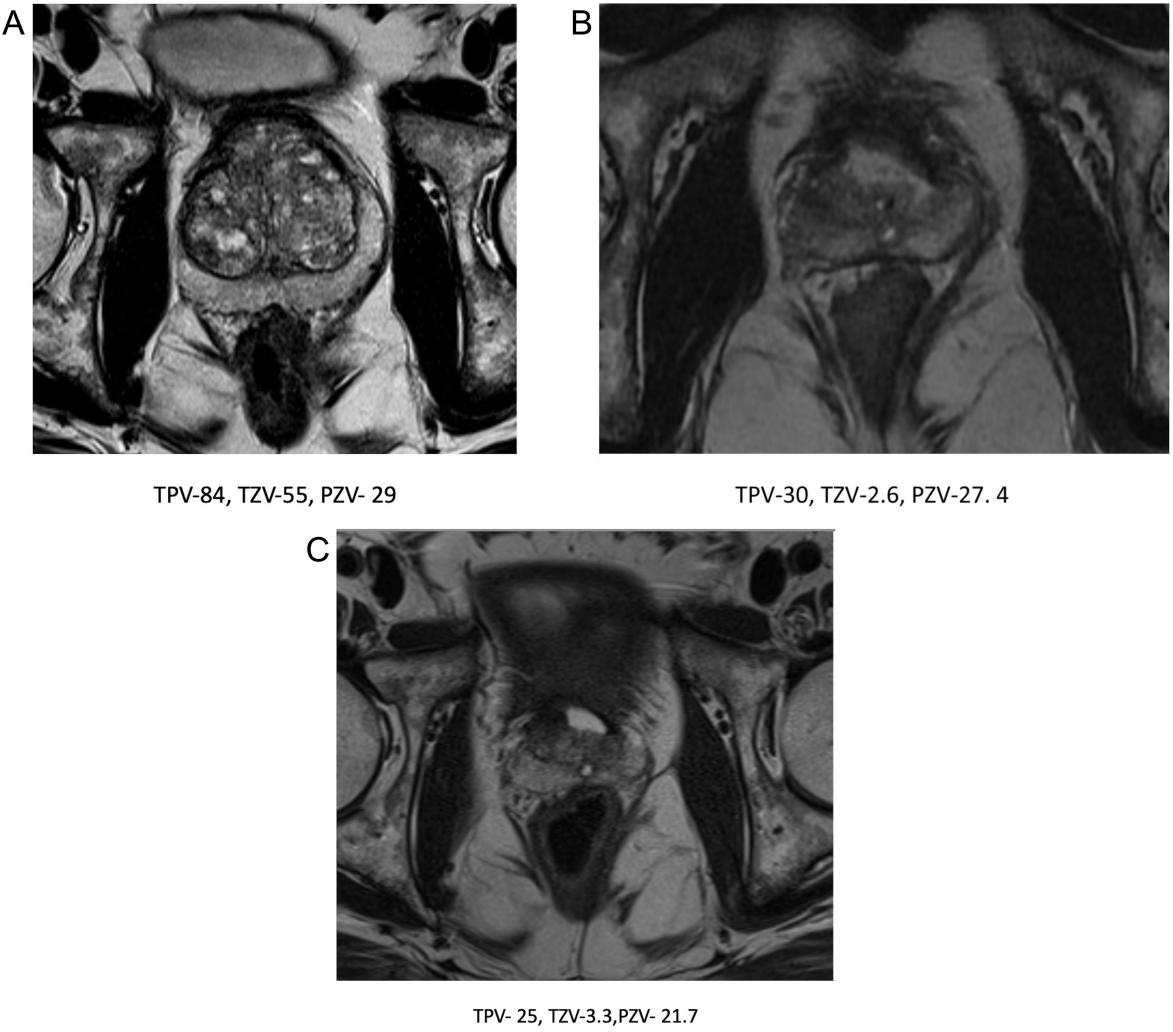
a-c. T2 weighted axial MRI images before and 6- week and 3 year after TURP procedure (volume in cc).
